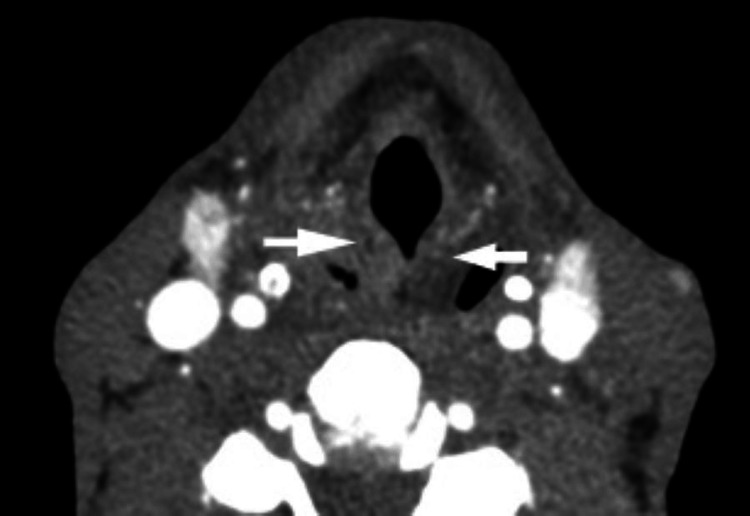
Neck CT scan of patient one The image shows bilateral symmetrical soft tissue thickening of the aryepiglottic folds (white arrows), up to 1.0 cm in diameter, without any focal enhancement mass being noted, and no enlarged cervical nodes were present.
